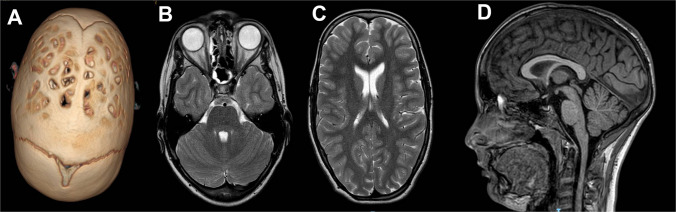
Preoperative 3D CT scan (A) of a young boy undergoing mini-invasive surgery at the age of 4 months. Postoperative MRI (B–D), performed 4.5 years later because of papilledema, does not show relevant findings
